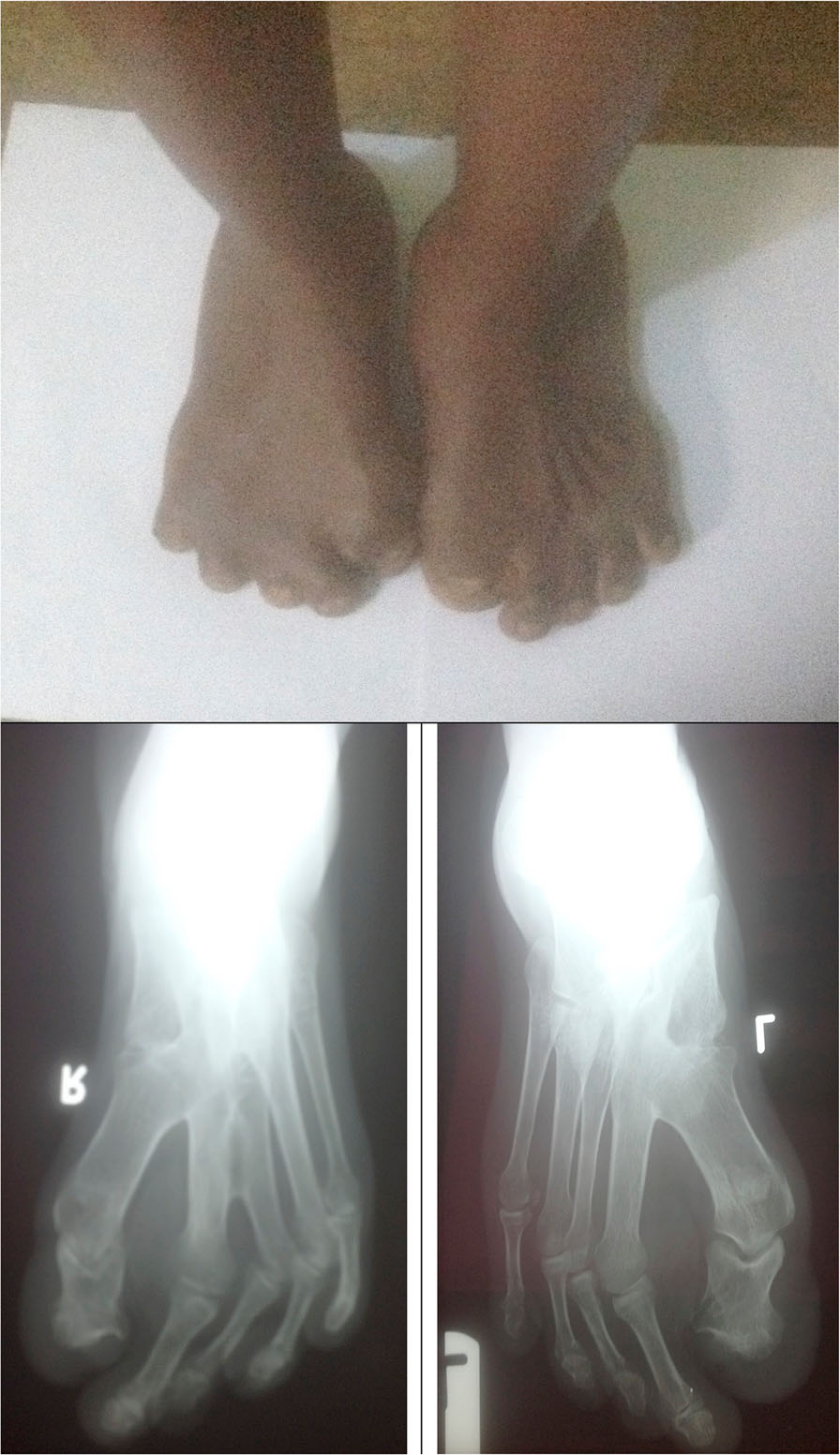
Picture and X-ray of the feet
